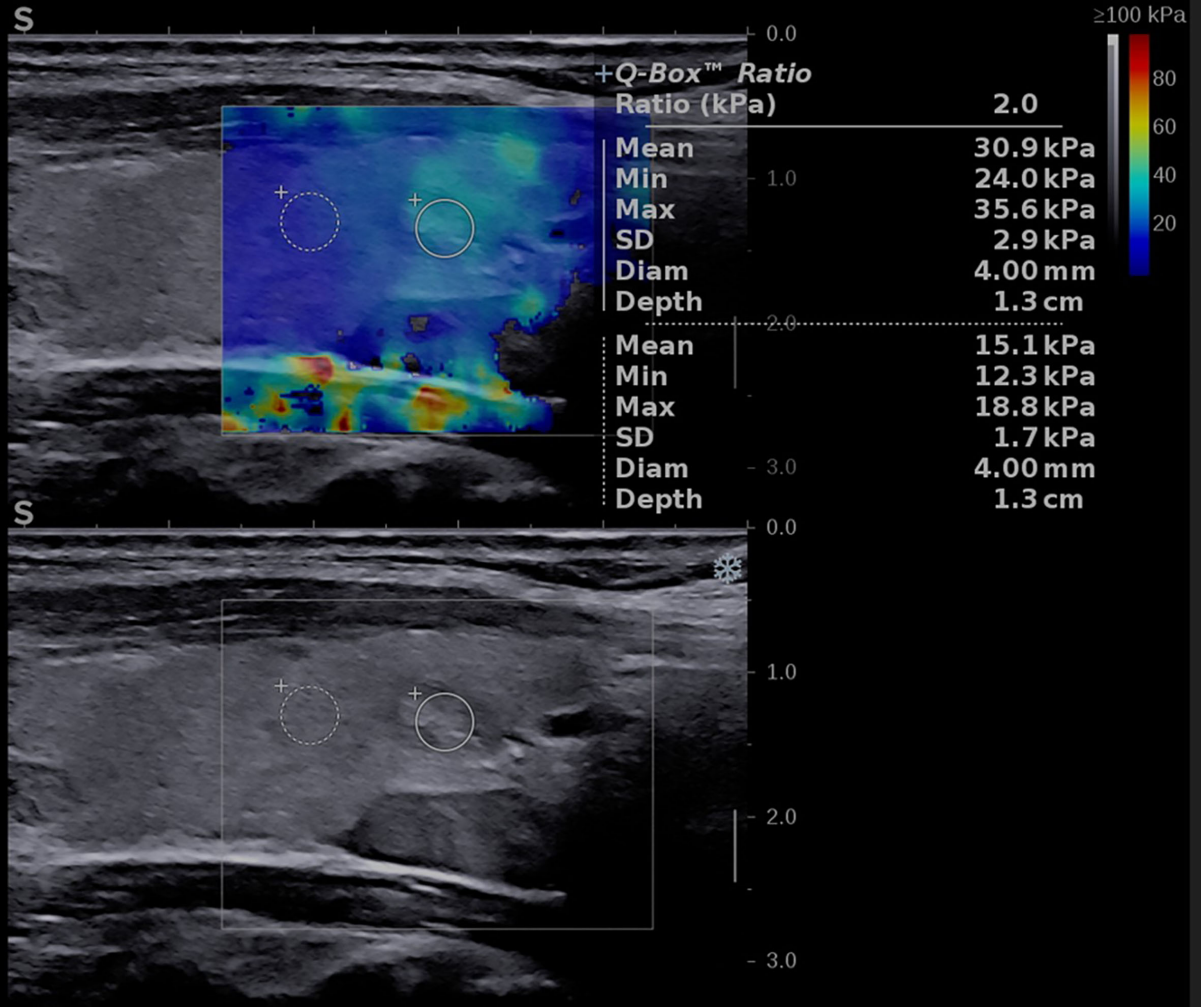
Conventional ultrasound image (displayed in the lower part of the image) showing an oval-shaped, well-delimitated, solid, inhomogeneous mass located in the left thyroid lobe. Shear-wave elastography image (shown in the upper part of the image) identifying a soft lesion, with a mean elasticity index (EI) of 30.9 kPa.
